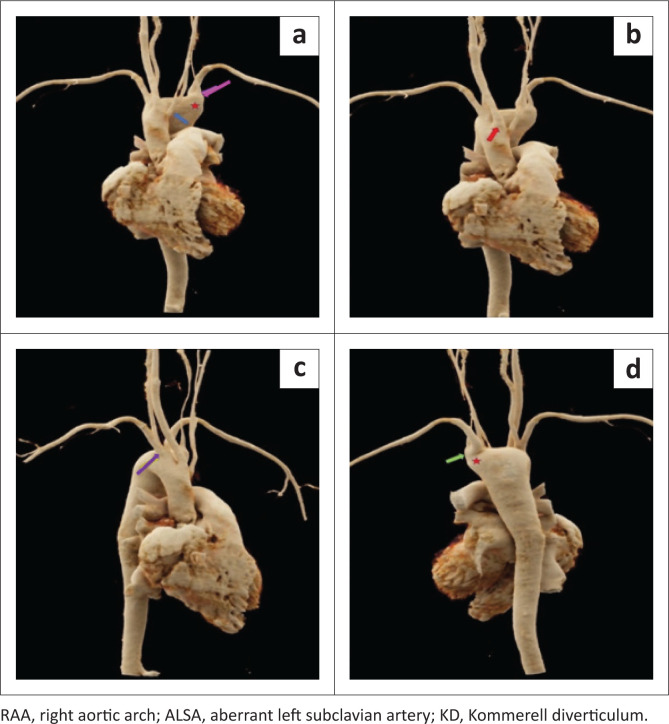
(a, b, c, d) 3D volume-rendered CT angiography of the thoracic aorta demonstrates a RAA with the left common carotid artery (blue arrow) originating as the first branch, followed by the right common carotid artery (red arrow) and the right subclavian artery (purple arrow); The ALSA (green arrow) originates from the KD (red star) as last branch. The left vertebral artery (pink arrow) is also seen arising from the KD.
